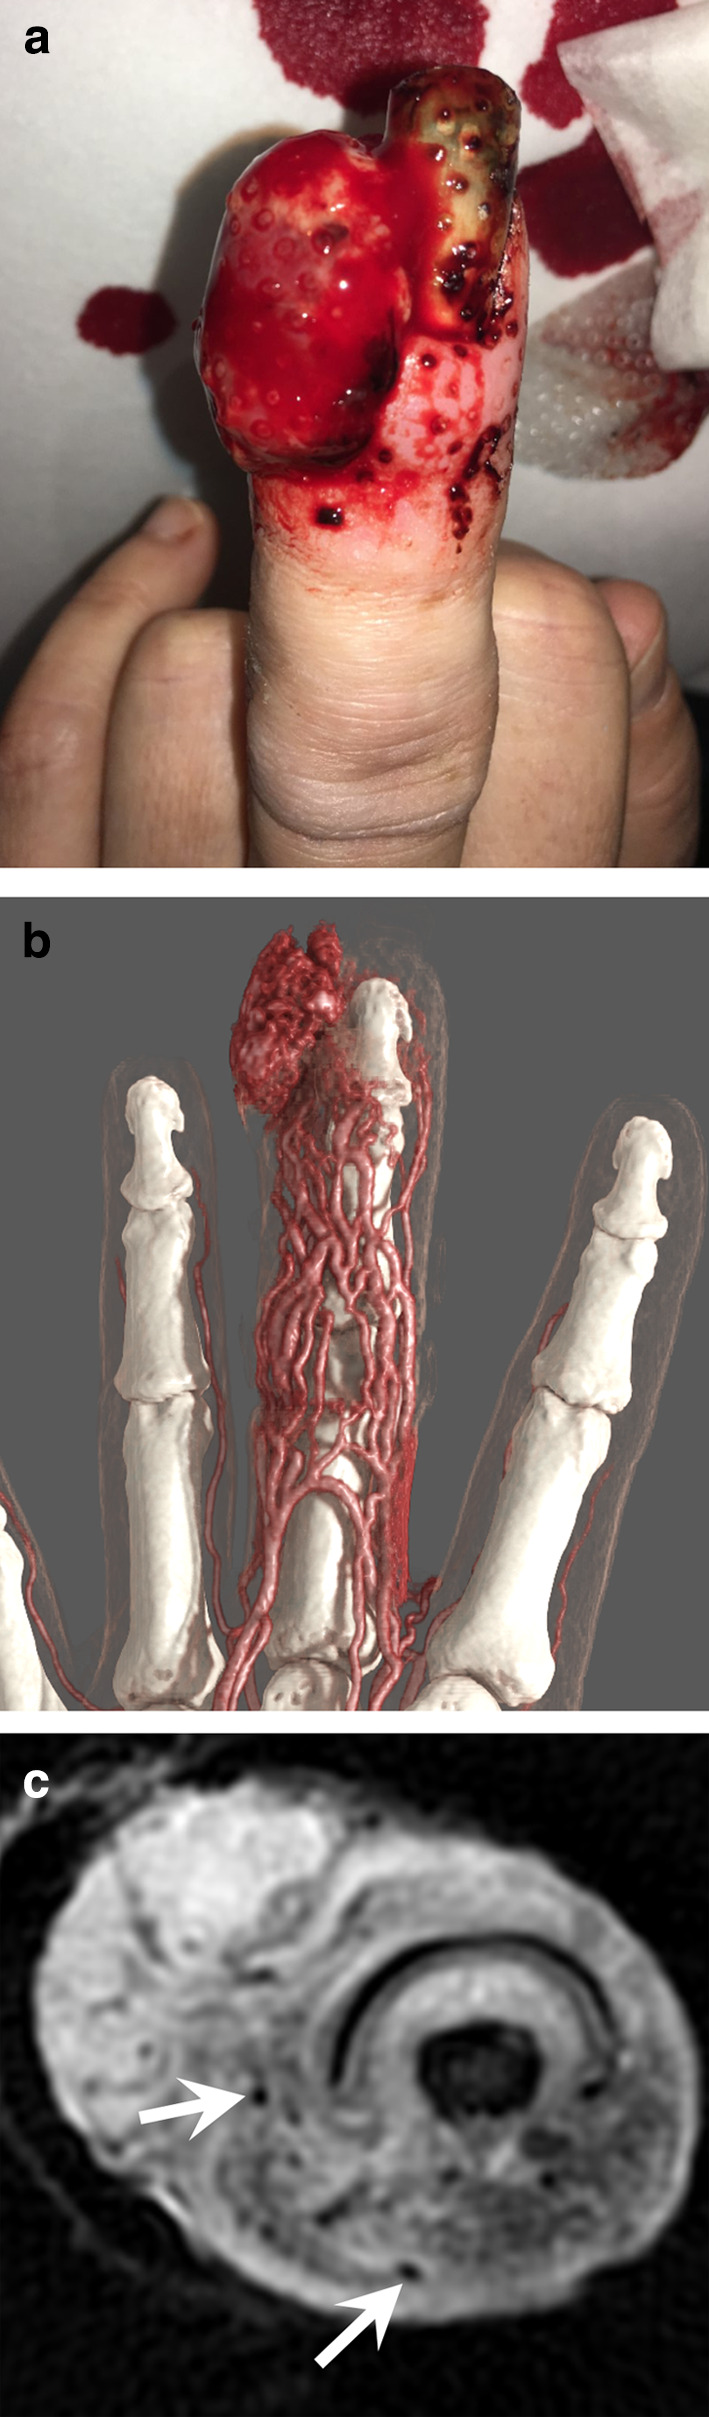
Pyogenic granuloma of the third finger in a 39-year-old woman who recently gave birth. a Photograph showing fleshy vascular, haemorrhagic exophytic tumour of the distal phalanx, and an enlargement of the finger. b Dynamic CTA showing an increased vascularity of the entire finger associated with a hypervascular exophytic soft tissue tumour. c Axial T2-weighted MR image showing an exophytic tumour without clear margins associated with oedema and vessels dilations with flow void (arrows) in the soft tissues
